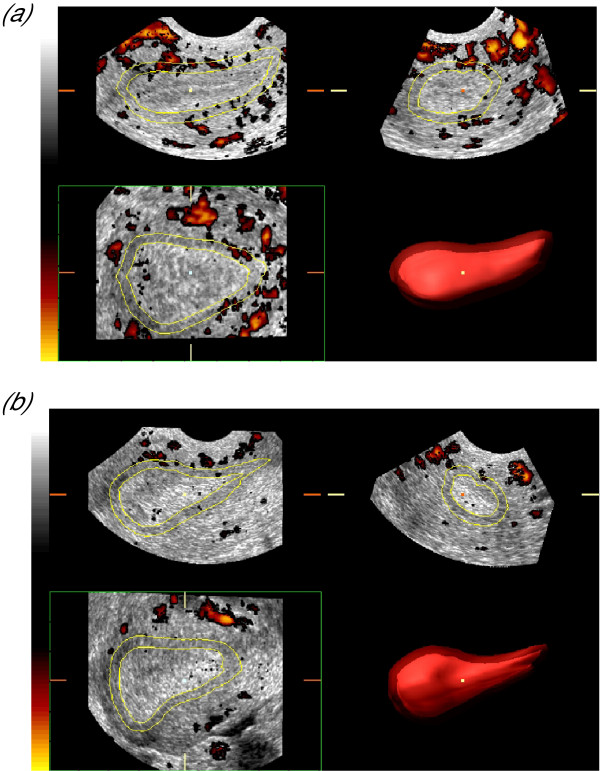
Intra-subject variation in endometrial blood flow. The degree of power Doppler information seen within Figure 6a is clearly superior to that seen in Figure 6b and this difference is quantifiable through the 'histogram' facility. These images also serve to demonstrate how vascularity is independent of morphometry and varies throughout different phases of the menstrual cycle as the less vascular homogenous endometrium characteristic of the luteal phase in Figure 6b is of greater volume than that in Figure 6a, which is the same endometrium at the end of follicular phase.
